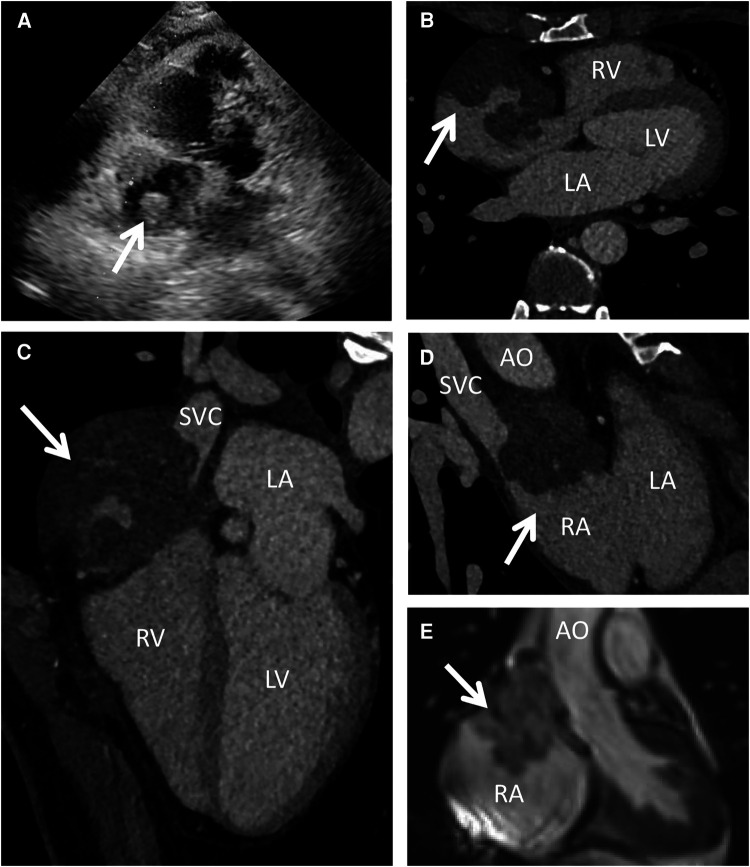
Preoperative echocardiography, cardiac CT and MRI. Echocardiography (A) showed multiple mixed echogenic masses on the lateral wall of the right atrium; CT scan (B–D) showed a giant cardiac mass with the invasion of the RA and SVC; MRI (E) indicated that the mass attached to the wall of RA with unclear boundary. AO, ascending aorta; LA, left atrium; LV, left ventricle; RA, right atrium; RV, right ventricle; SVC, superior vena cava.
